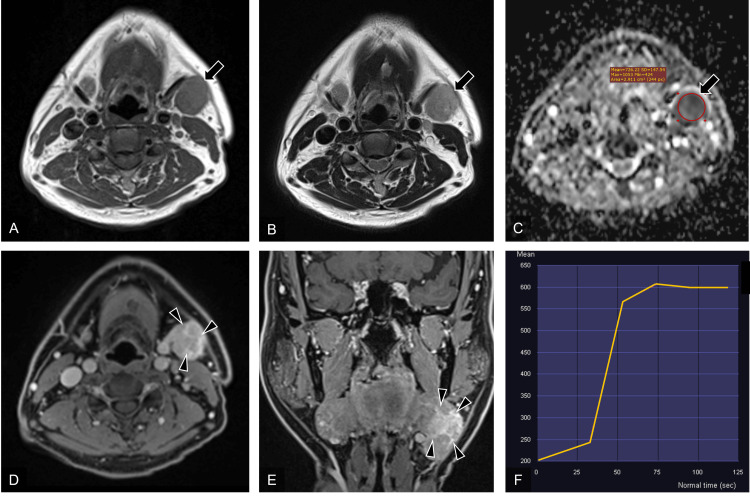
Magnetic resonance images of the mass of the left submandibular gland. T1WI: T1-weighted image; T2WI: T2-weighted image; ADC: apparent diffusion coefficient; Gd-FS-T1WI: gadolinium-enhanced fat-suppressed T1WI; DCE-MRI: dynamic contrast-enhanced magnetic resonance imaging (A) axial T1WI, (B) axial T2WI, (C) ADC map derived from diffusion-weighted images (b = 1000 s/mm^2^), (D) axial Gd-FS-T1WI, (E) coronal Gd-FS-T1WI, (F) time-intensity curve of DCE-MRI. The mass shows moderate signal intensity on T1WI (A, arrow), a slightly high signal intensity on T2WI (B, arrow), and low signal intensity on the ADC map (C, arrow). The ADC value was 0.726 × 10^-3^ mm^2^/s (C). The time-intensity curve shows rapid initial enhancement before reaching a plateau (F). The mass shows internal septal-like enhancement (D, E, arrowheads).
